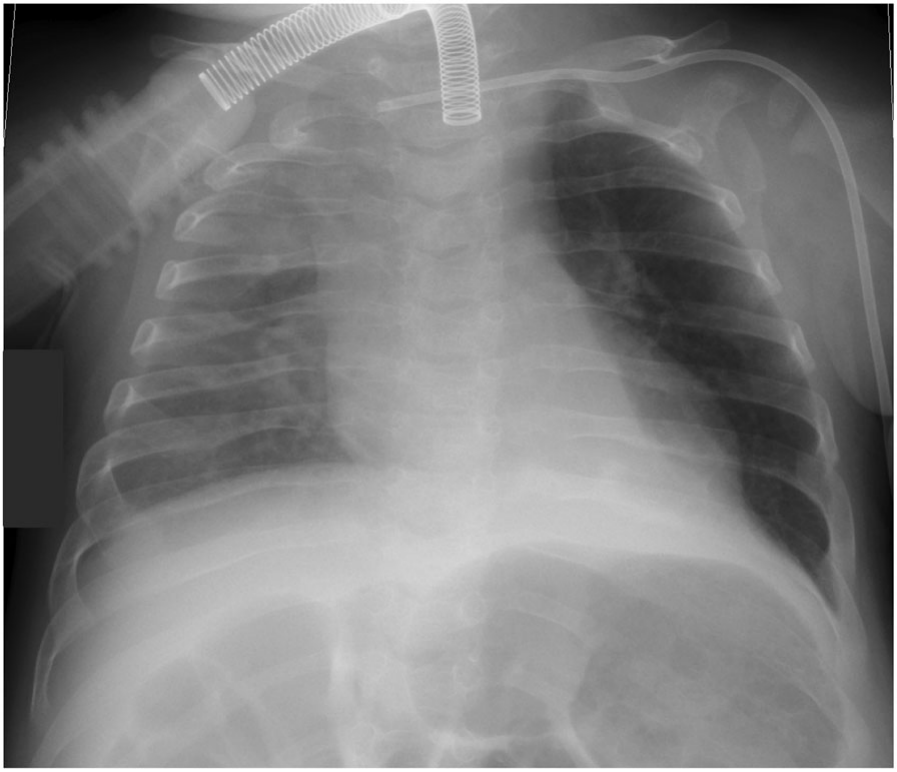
Chest radiograph of Patient 1 after 11 months of treatment with asfotase alfa. Chest radiograph of Patient 1 at 1 year of age, after 11 months of treatment with asfotase alfa. The radiograph showed an improvement in the mineralization of the ribs and an increase in the volume of the thoracic cavity compared with that observed at age 4 weeks (see Fig. 1b)
